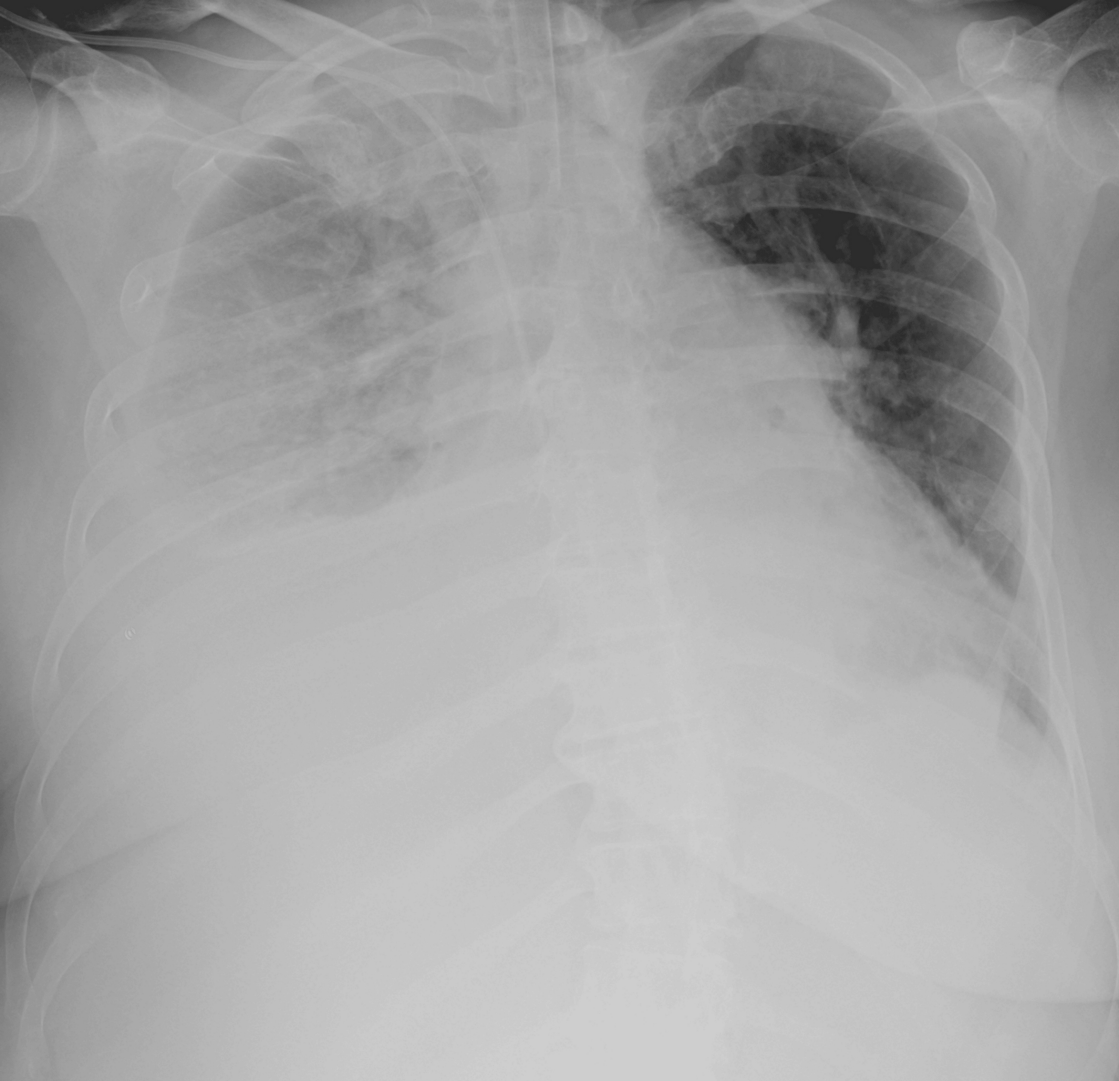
Chest X-ray - pleural effusion with confluent pulmonary infiltrates
